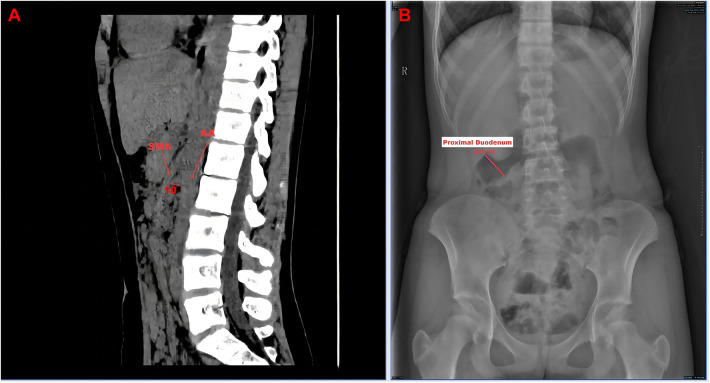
Preoperative imaging examinations. (A) CT scan depicting a decreased angle between SMA and AA. (B) X-ray demonstrating dilation of the proximal duodenum.
